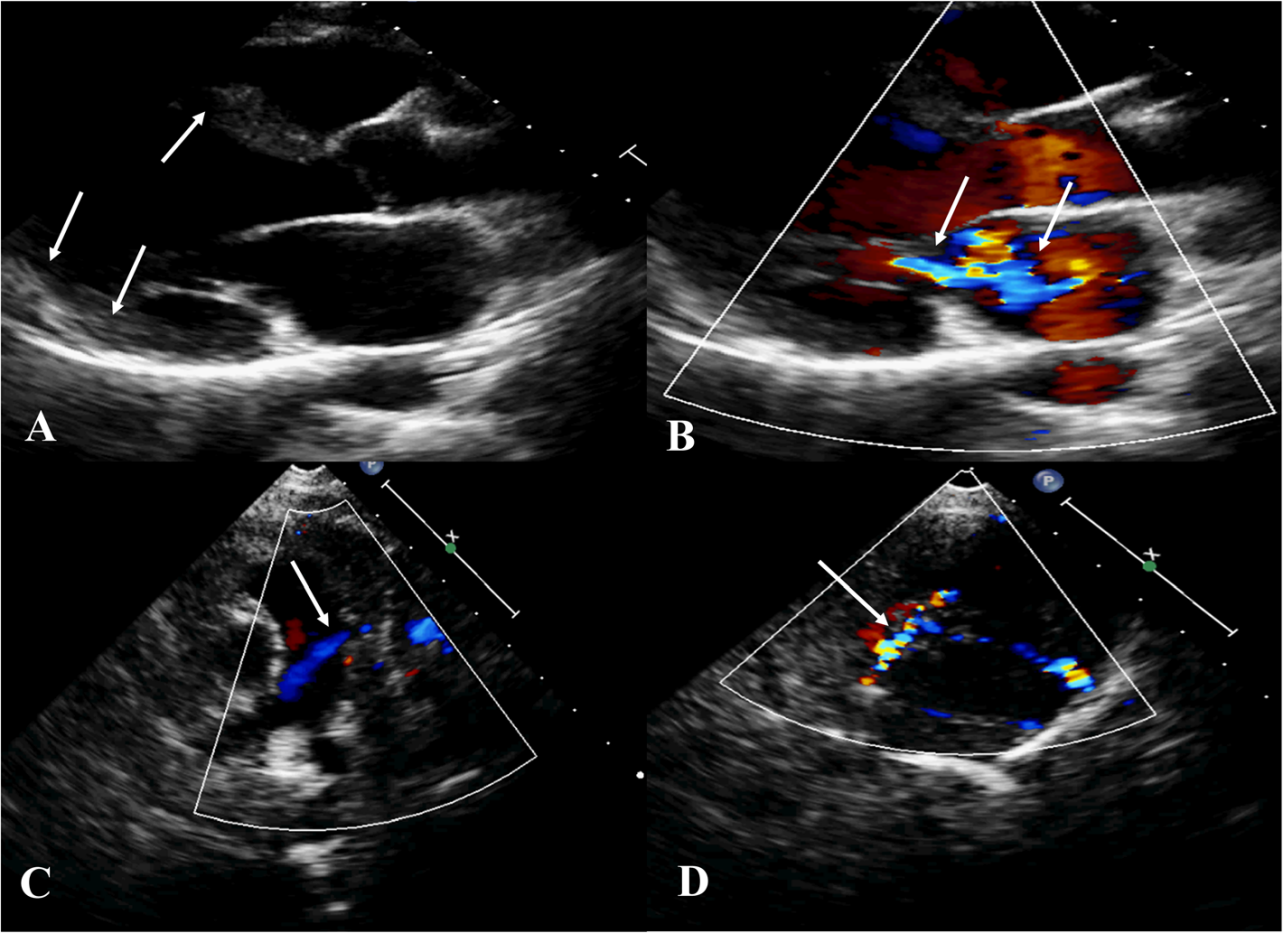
a 2D on parasternal long axis view. White arrows show the dilated left ventricle (LV). b Color 2D on parasternal long axis view. White arrows show Mitral regurgitation (MR). c Color 2D on parasternal short axis view. White arrow shows the retrograde flow from the left coronary artery (LCA) into the pulmonary artery. d Color 2D on parasternal short axis view. White arrow shows the increased flow in the intraventricular collateral vessels from the RCA to the LCA
